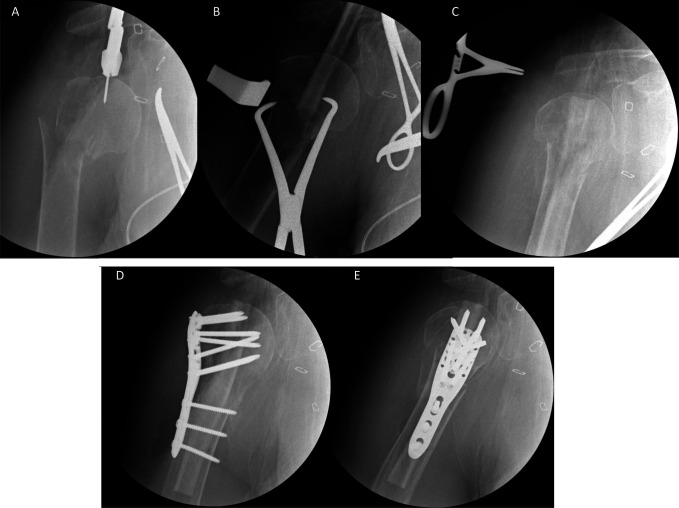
Radiograph demonstrating the starting point that is localized at the cranial aspect of the humeral head with a 2.0-mm Kirschner wire (A). The wire is advanced approximately 1-cm. A reamer is positioned over the wire and manipulated by hand to create the entry portal for the fibular strut (A). The strut is advanced through the humeral head; on advancing it to the fracture site, a reduction is achieved (B) to facilitate passage into the distal segment (C). Standard lateral locking plate fixation is thereafter applied (D and E).
